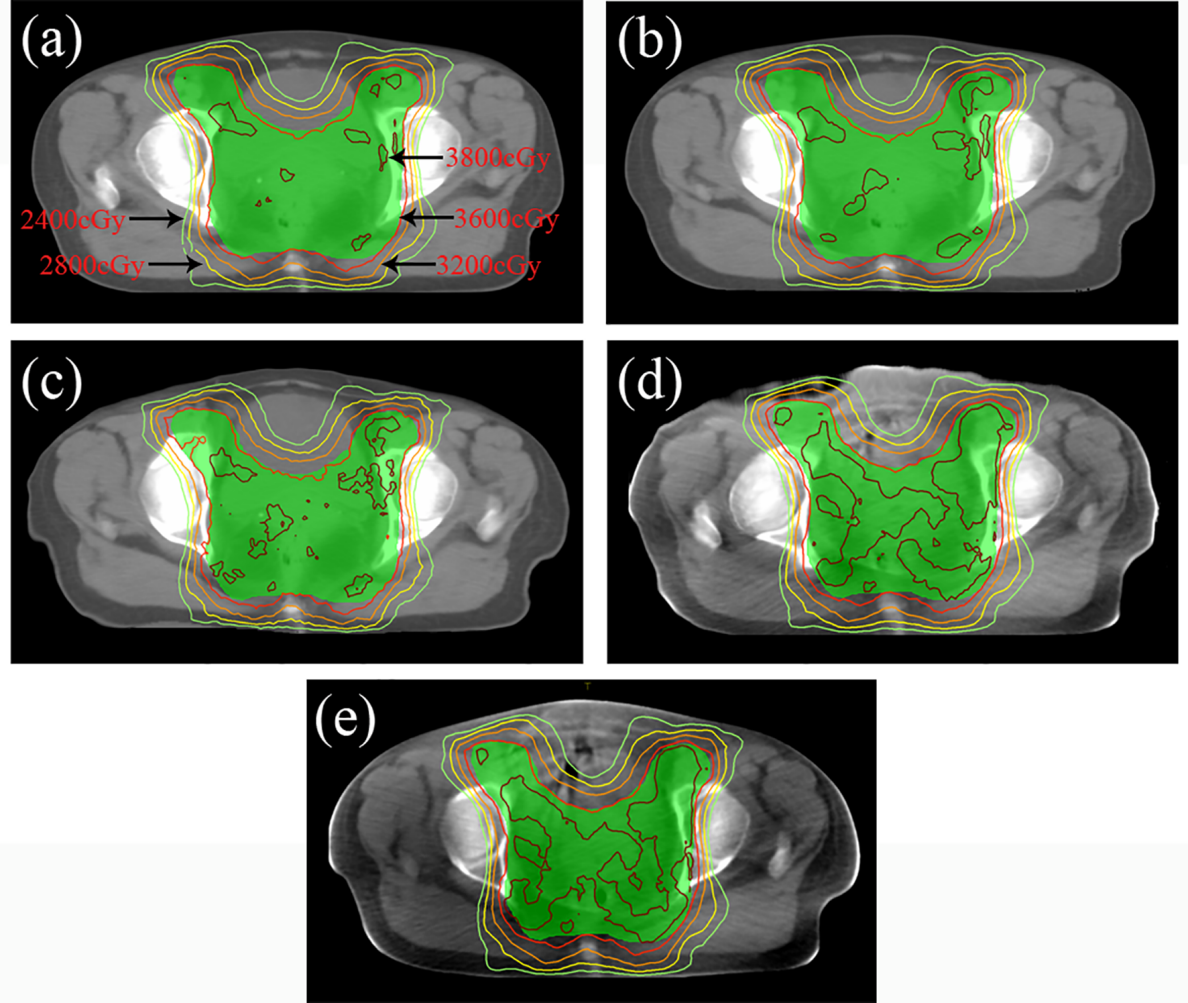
Dose distribution of radiotherapy plan based on four CT images at the isocenter level. (A) Dose distribution of CT_gt_ at isocenter. (B) Dose distribution of CT_CycleGAN_ at isocenter. (C) Dose distribution of CT_unet-GAN_ at isocenter. (D) Dose distribution of CT_FCN-GAN_ at isocenter. (E) Dose distribution of CBCT at isocenter.
